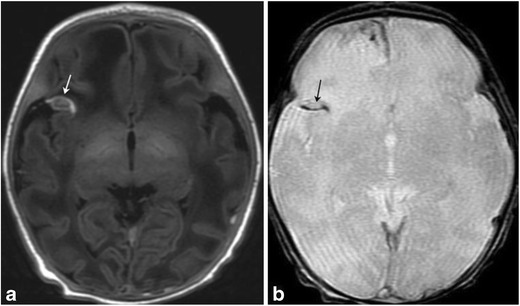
Subarachnoid hematoma Axial T1/GRE MRI in a 9-day-old term neonate with history of difficult, vacuum-assisted delivery. Right frontal blood is noted in a gyriform distribution, suggesting subarachnoid blood. Subdural blood was also noted tracking along the falx
